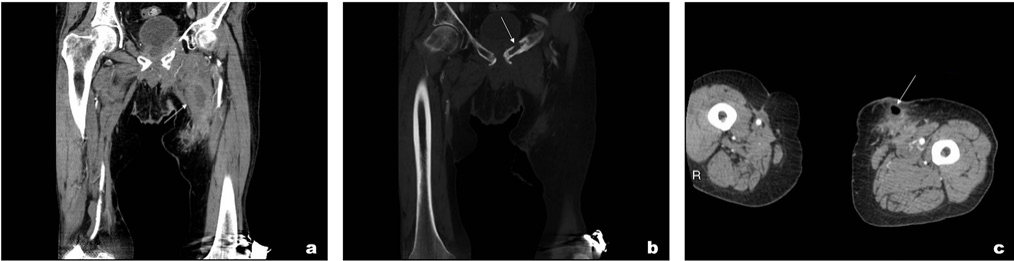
(a) Post-contrast CT coronal slice showing a thick-walled collection involving the left adductor longus & brevis with small fluid pockets surrounding the pubic symphysis. (b) Bony window shows destruction of the pubic symphysis. (c) Post-contract CT axial slice showing gas pockets within the subcutaneous tissue with surrounding fluid and fat stranding.
